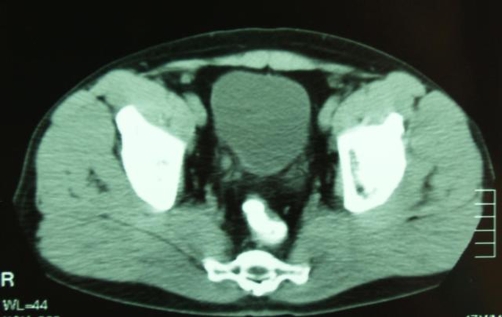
Preoperative CT imaging showing a lesion of the lower one third of the right ureter in close proximity to the bladder wall.
